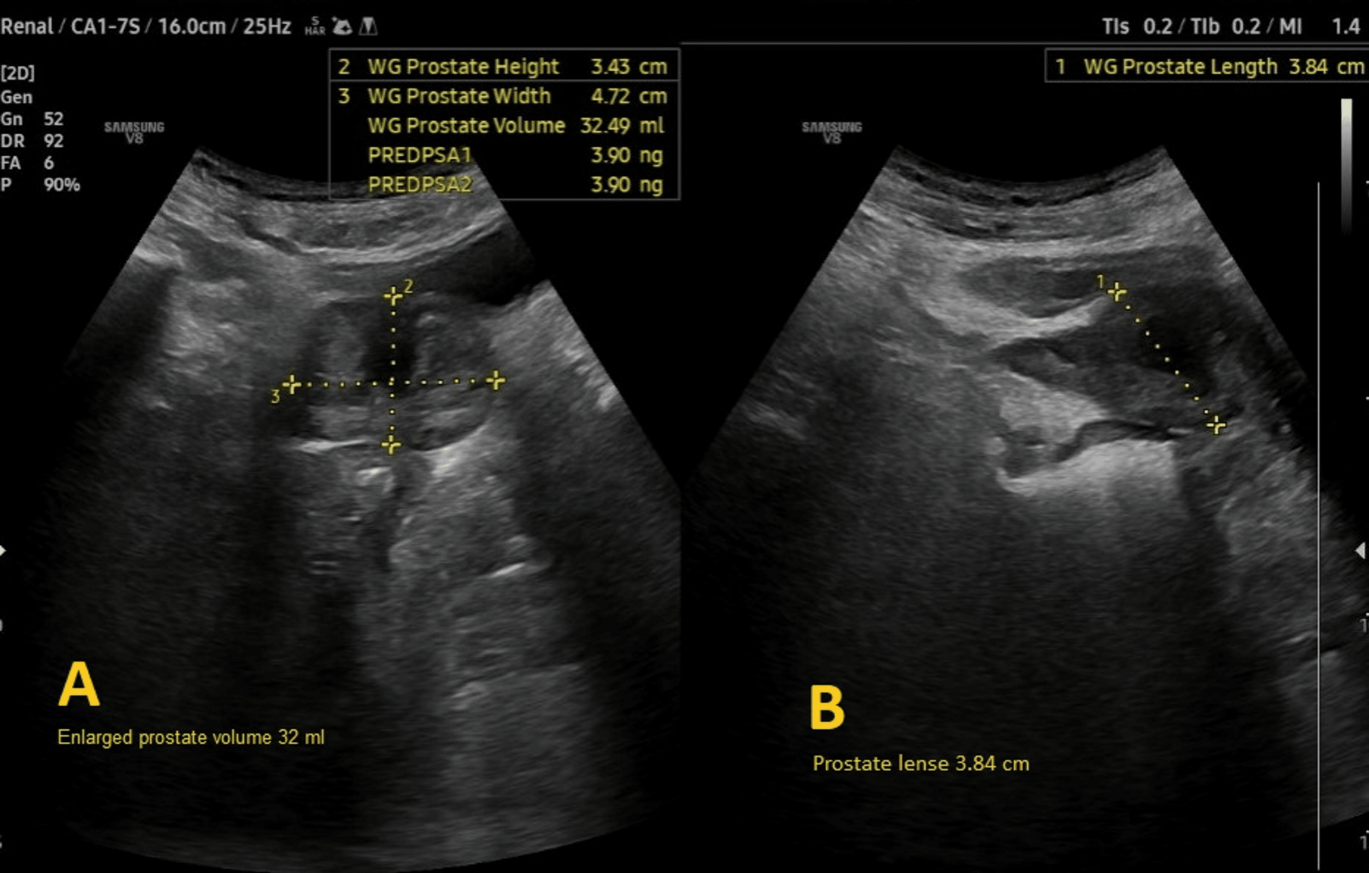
Pelvis ultrasound images showing an enlarged prostate.
